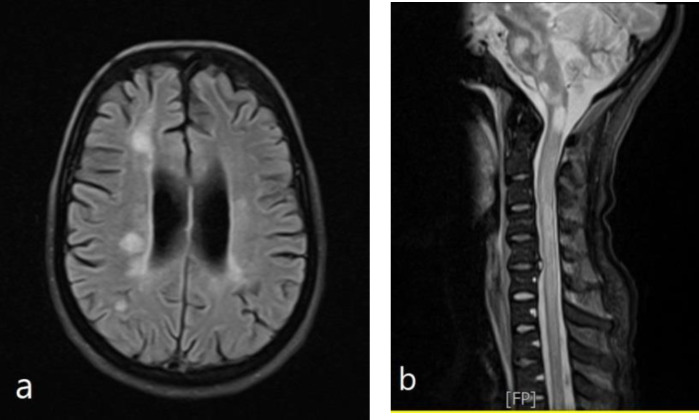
Axial and sagittal views of the brain and cervical MRI of the second case
